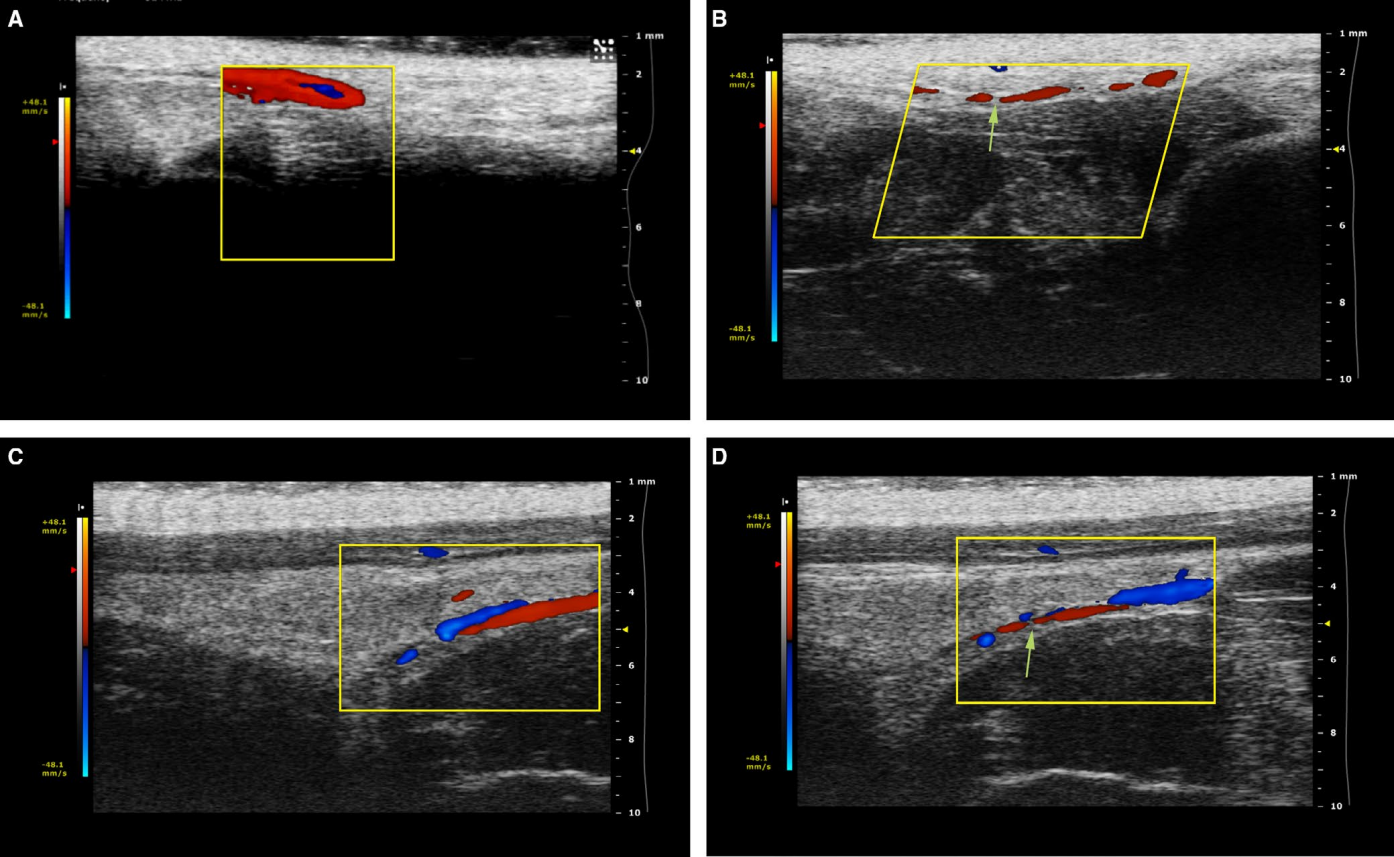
Results of colour Doppler ultrasonography demonstrated blood flow in different time points. A, Normal vessel, arterial blood (red) and venous blood (blue) flow; B, Four weeks after balloon‐induced endothelial injuries, and apparent discontinuation of arterial blood flow was observed (atherosclerosis damage was formed); C, Arteries recanalization of atherosclerosis plaque by ercutaneous transluminal angioplasty (PTA), and obstructed arterial blood vessel returned to recovery; D, Four weeks after PTA, iliac arterial blood flow blocked again and the restenosis plaque was established. When checking the arteries, the blood flow is directed towards the ultrasound probe. When checking the veins, the blood flow is facing away from the probe. Dashed lines indicated the position of the iliac artery. The yellow arrow indicated the formation of plaques and obstructed arterial blood flow, and scales were shown in the rightmost column of the image in red frame
